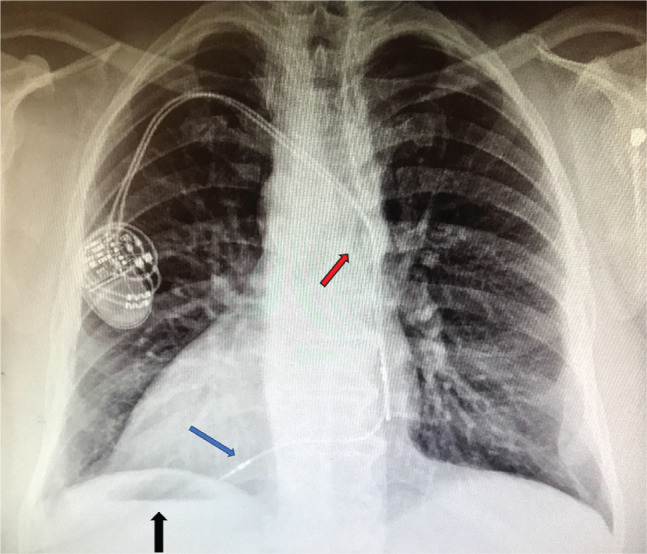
Chest X-ray showing DX-SIT. The black arrow points to a gastric bubble under the right hemidiaphragm. The red arrow points to the atrial and ventricular leads in the left-sided SVC. The blue arrow points to the ventricular lead.
